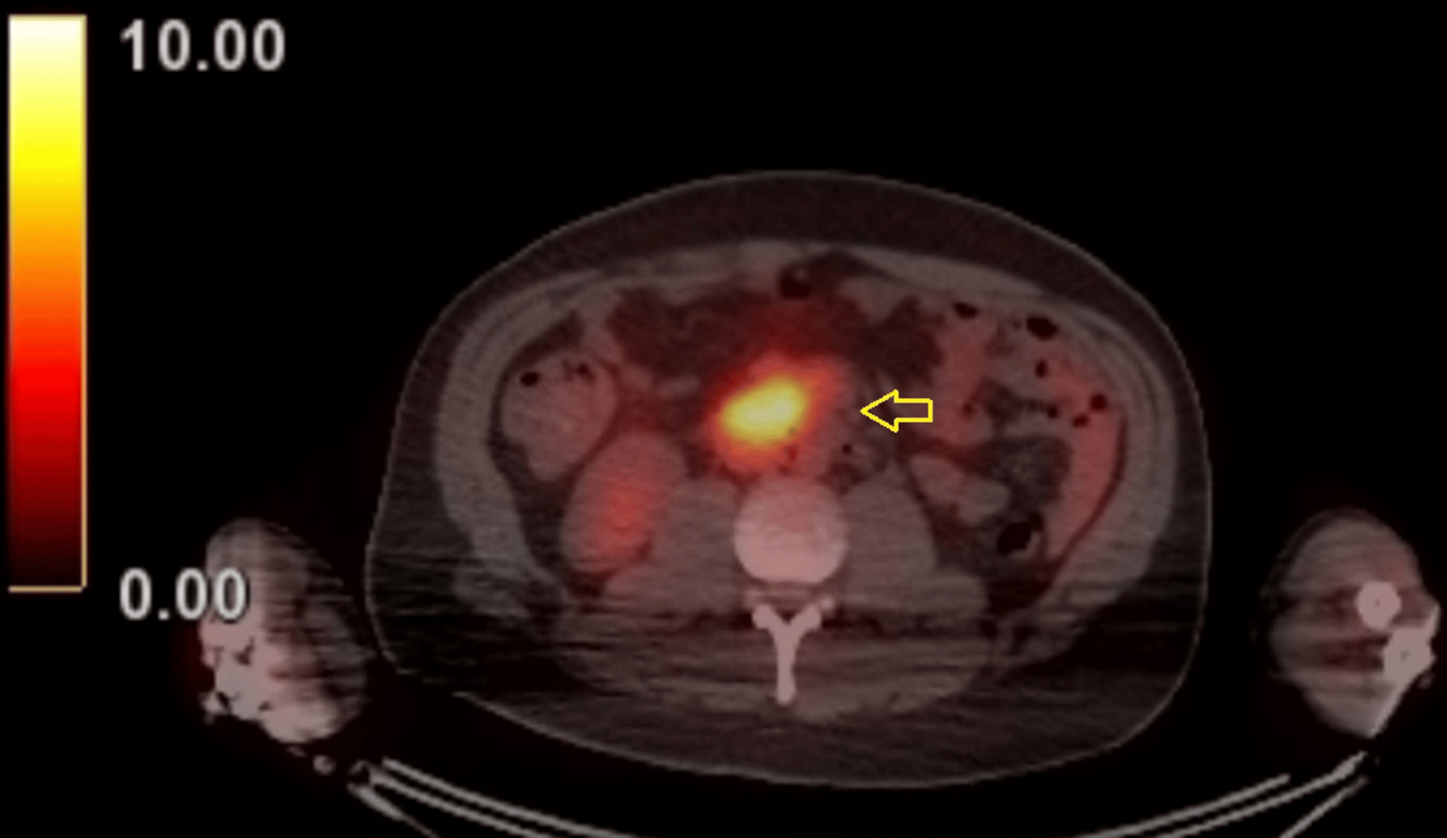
PET-CT scan demonstrating intensely FDG avid central mesenteric lymph node conglomerate (arrow) measuring approximately 2.9 x 2.3 cm in maximal transaxial dimension, SUV 5.6. PET-CT, Positron emission tomography combined with computed tomography; FDG, fluorodeoxyglucose; SUV, standardized uptake value.
